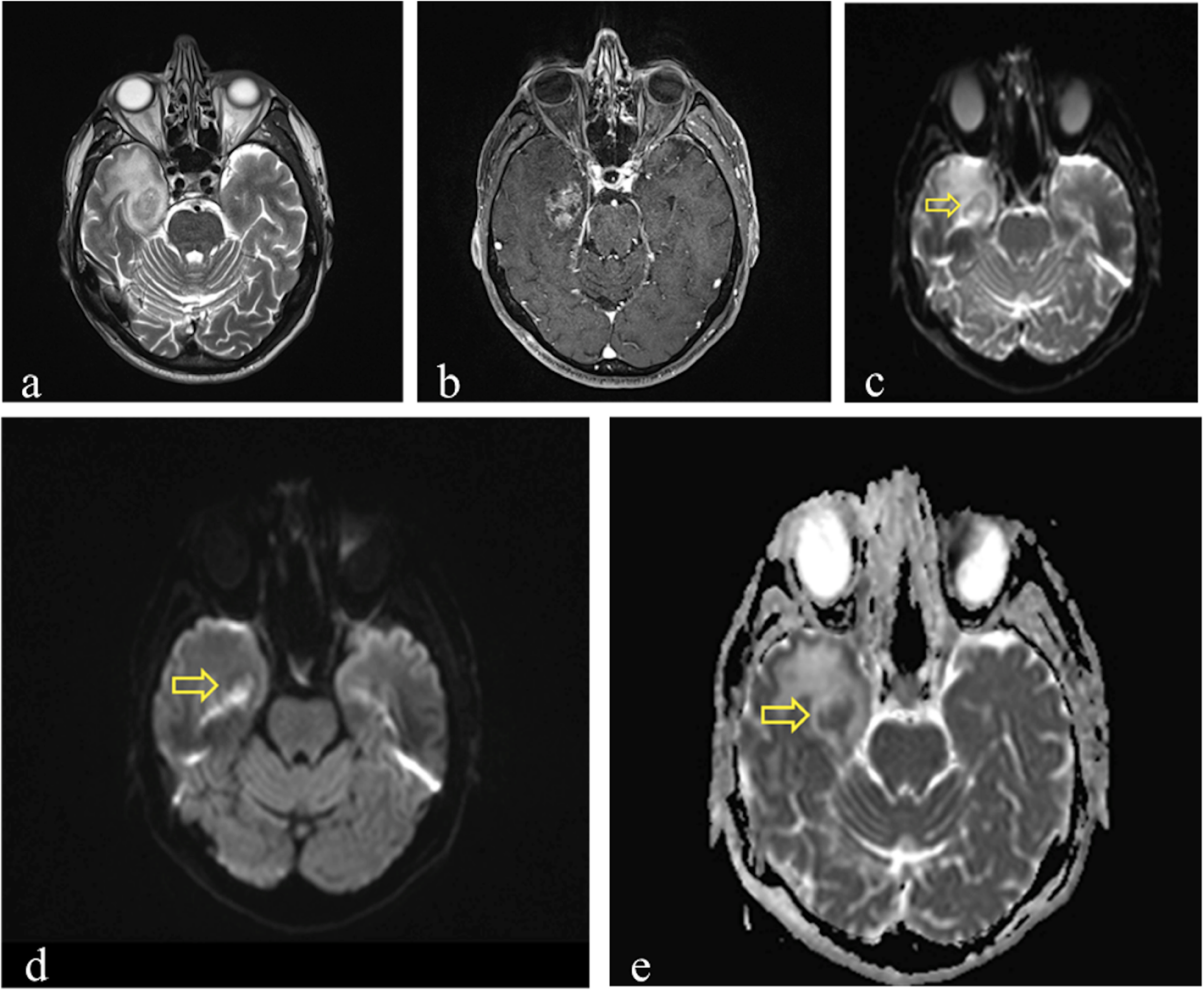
MRI performed 18 months after 65.6 Gy carbon ion therapy in a patient with sinonasal adenoid cystic carcinoma. Axial T2-weighted image (a) shows a subcortical white matter and gray matter lesion in right polar and mesial temporal regions. Axial post contrast T1-weighted image (b) shows the presence of inhomogeneous contrast-enhancing lesion. Diffusion imaging documented the presence of foci of restricted diffusion within the medial part of the lesion appearing as hyperintense areas in DWI-*b0* (c) and DWI-*b1000* images (d) and hypointense on the corresponding ADC map (e) (yellow arrows)
